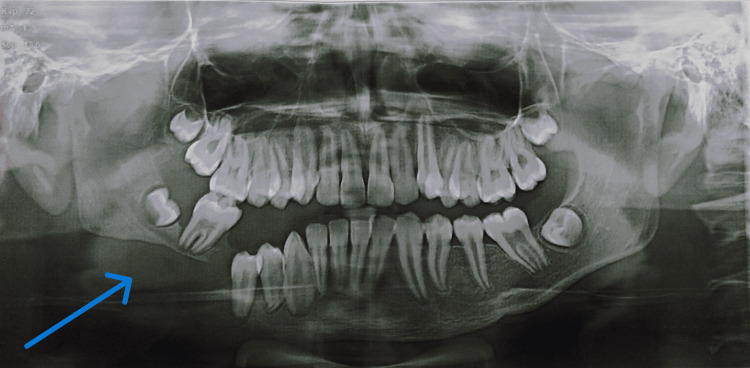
A panoramic radiograph revealed an excessively thin bone rim with a pathological fracture in the right body of the mandible.
